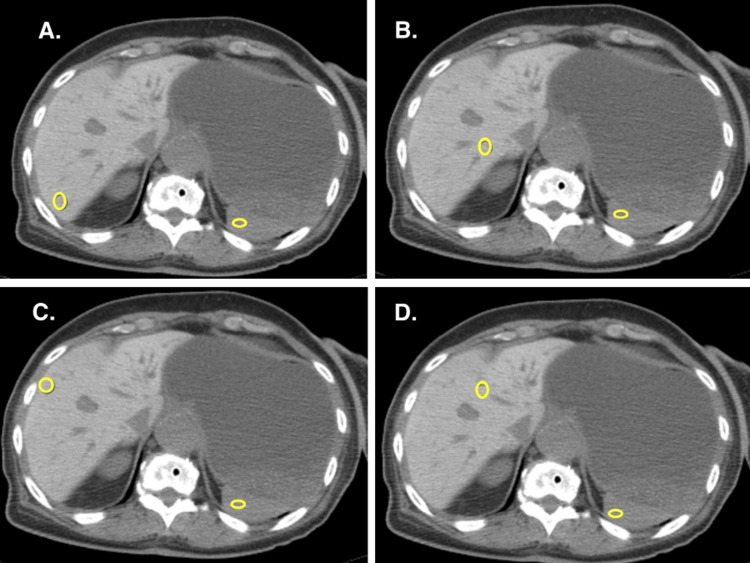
Non-contrast computed tomography (CT) of the abdomen with encircled regions of interest (ROI) in the liver and spleen A. Liver attenuation of 139 Hounsfield Unit (HU) and splenic attenuation of 44 HU at ROI B. Liver attenuation of 135 HU and splenic attenuation of 52 HU at ROI. C. Liver attenuation of 142 HU and splenic attenuation of 46 HU at ROI. D. Liver attenuation of 148 HU and splenic attenuation of 50 HU at ROI.
